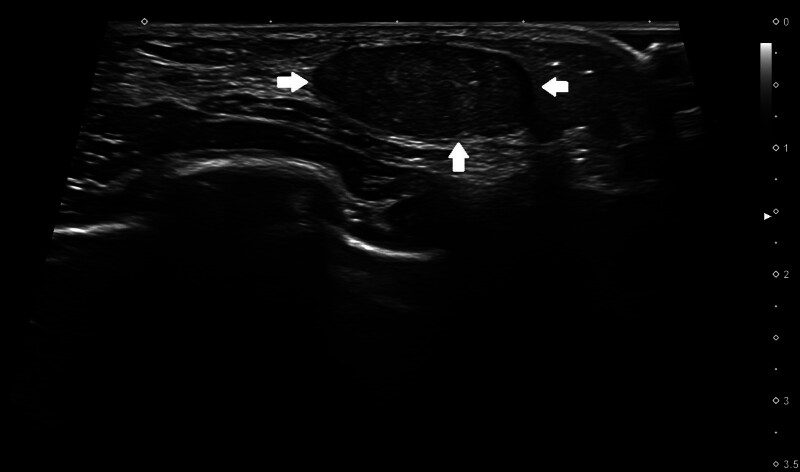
Ultrasound findings showing a well-defined mass measuring 1.7 cm × 1.7 cm × 0.7 cm (arrow), surrounded by a peripheral hypoechoic rim in the left breast.
